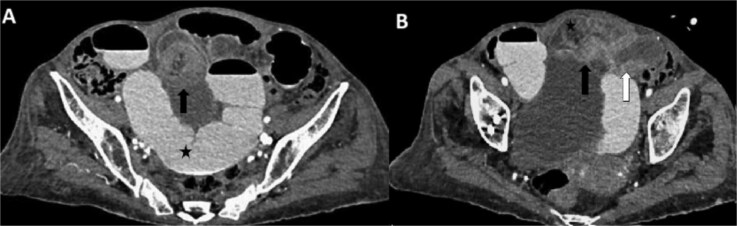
Abdominal CT scan with oral and intravenous contrast with intussusception features in axial view: (A). There is generalized dilation of the small intestine (black star) with contrast medium present within the lumen. At the ileal level, a rounded, heterogeneous tubular structure is noted, dependent on the loops of the small intestine, exhibiting a ‘target sign’ (black arrow) alongside increased wall thickness and edema. (B). Protrusion through another rounded mass, creating a ‘bowel-within-bowel’ appearance, characterized by an amorphous lesion acting as a lead point (black arrow) arising from the distal ileum (white arrow), which is causing intussusception in the proximal ileum (black star).
